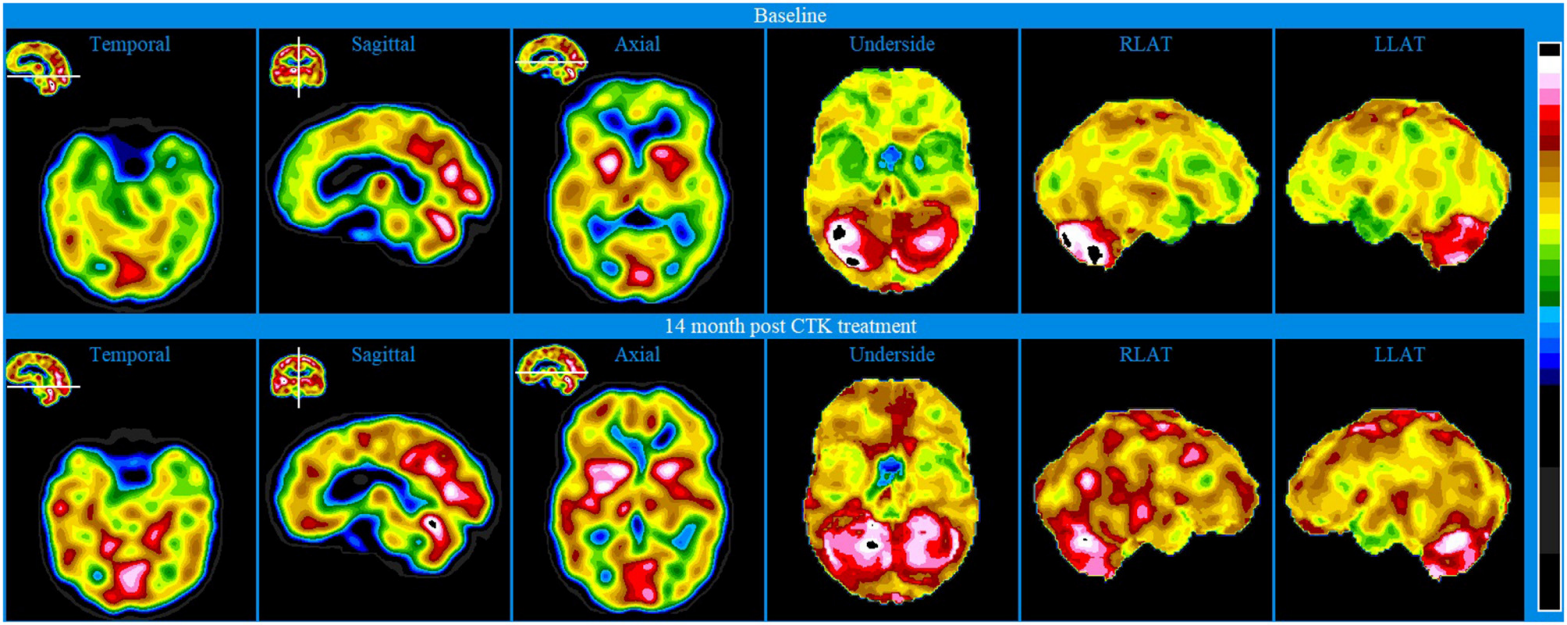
Brain SPECT images for Patient VI. Top line relates to brain SPECT images at baseline. Bottom line shows SPECT images 5.5 months following 24 sessions of CTK treatment. In each row (from left to right) one temporal slice, one sagittal slice and one axial slice and three stereotactic surface projections of the underside, right lateral (RLAT) and left lateral (LLAT).
